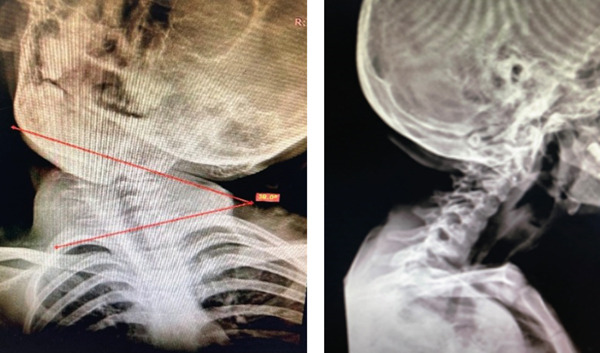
The preoperative anteroposterior and lateral plain radiographs show a 38^0^ cervicomandibular angle of cervical part of spine.
